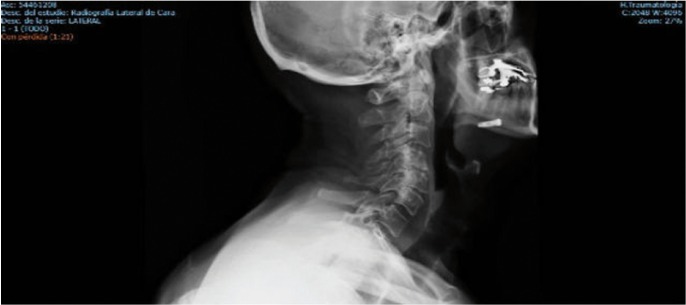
Implant displacement into sublingual space. Side view face X-ray.
